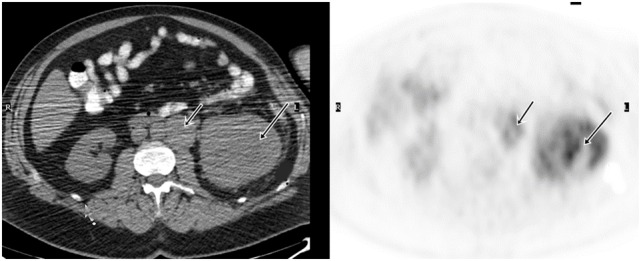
Demonstration of primary RCC and tumor thrombosis on FDG PET/CT. A 53-year-old man had a large left renal mass seen on the CT. FDG PET/CT showed increased, heterogeneous uptake of the mass in the left kidney. There was also tumor thrombosis in the renal vein, evidenced by FDG avid intraluminal lesion.
